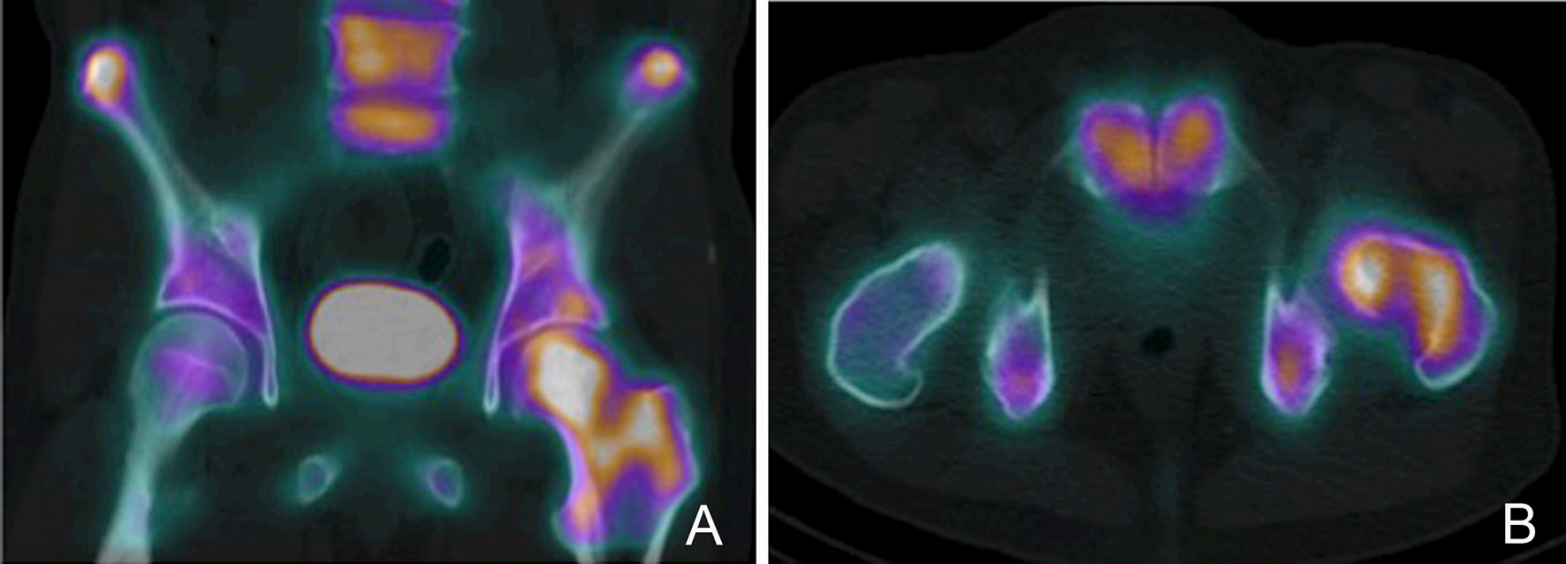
PET-CT scan demonstrating a left proximal femur metastatic lesion. (A) Coronal and (B) axial cuts of the PET-CT images demonstrating intense FDG uptake at the left femoral head, neck and intertrochanteric region.
